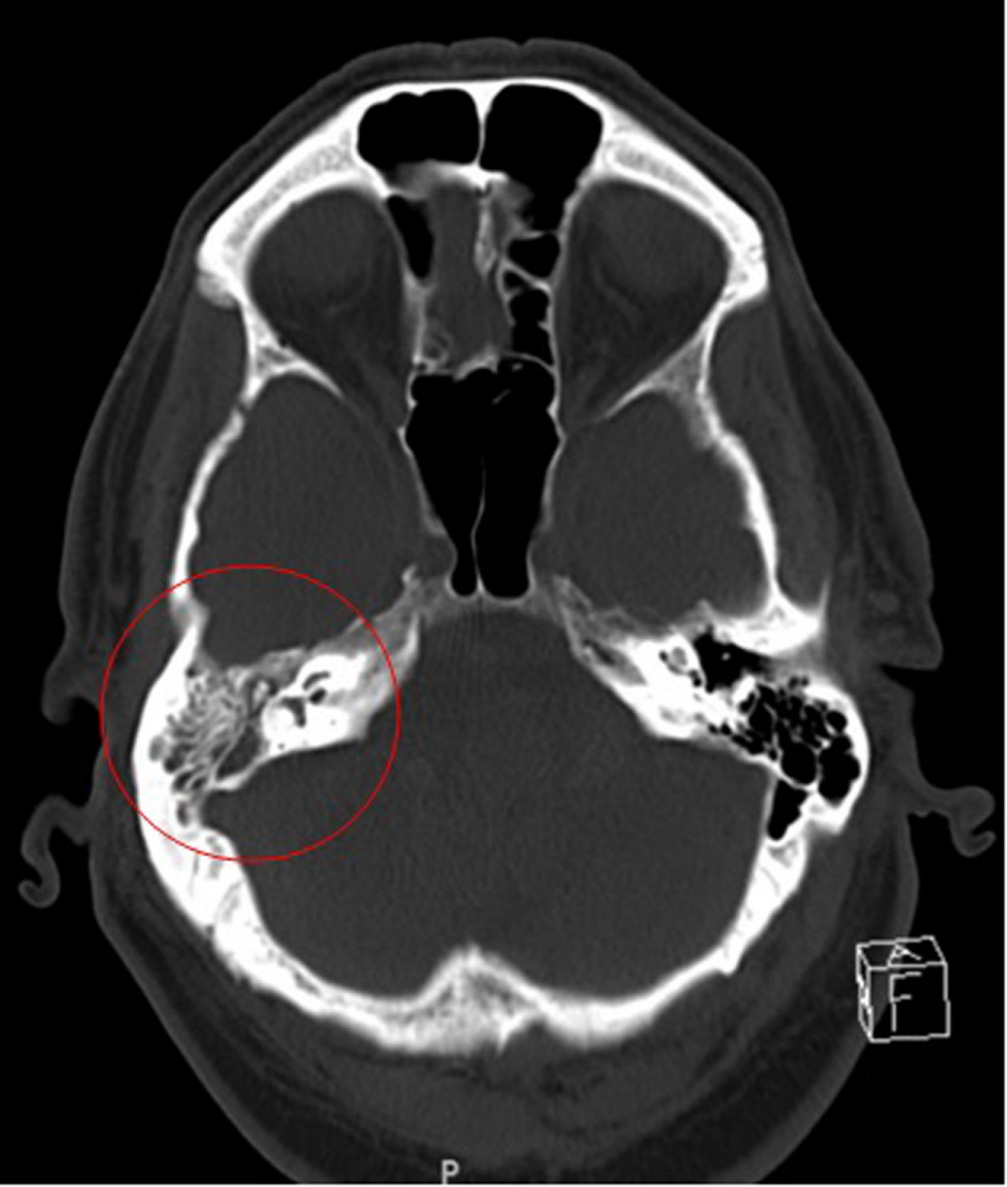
CT head without contrast revealing a right sided tympanomastoid effusion.
